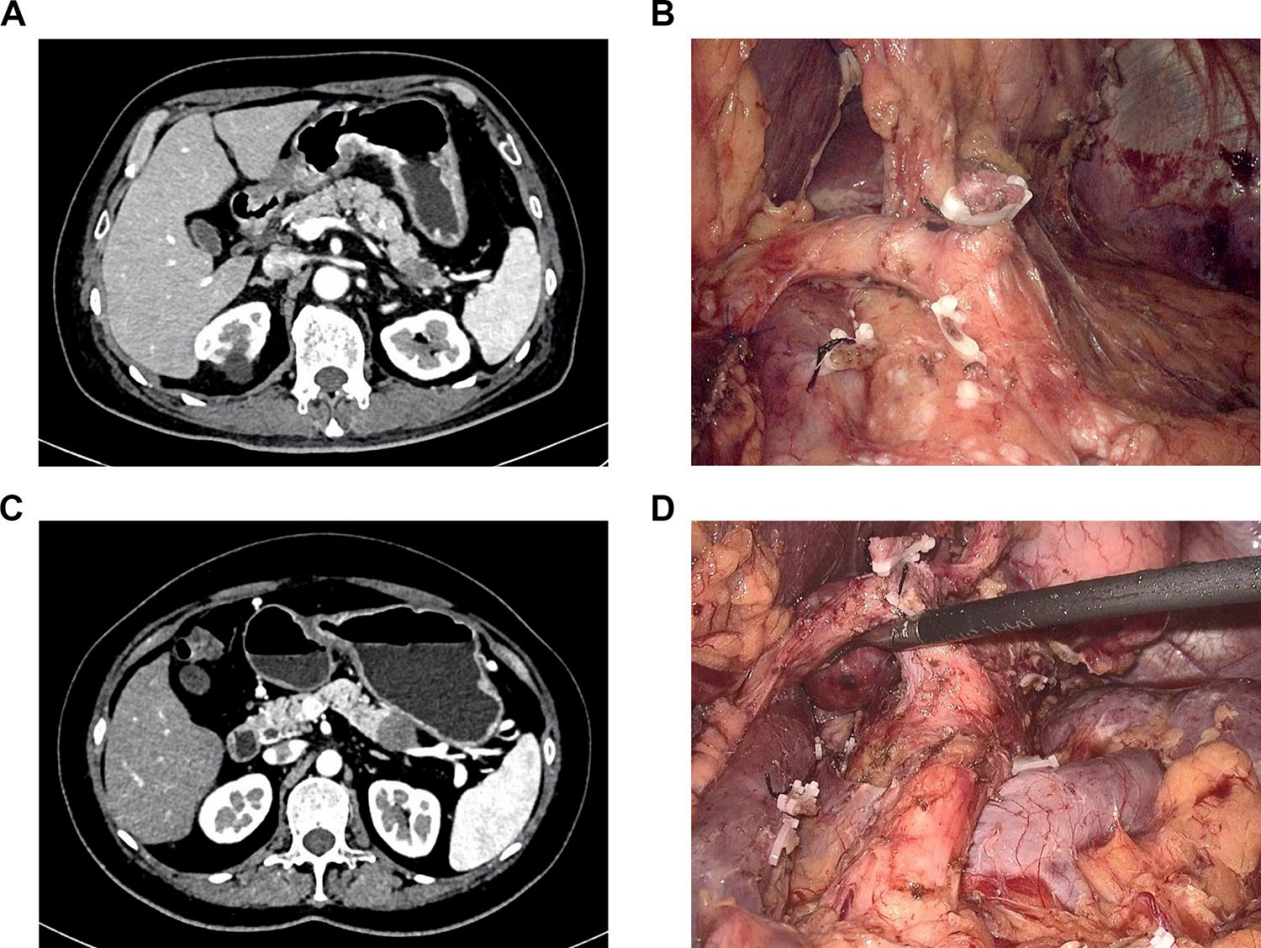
Preoperative CT images of left-sided PDAC meeting the selection criteria and the extent of surgical resection under laparoscopy. A and B Preoperative CT images (A) of a pancreatic tail cancer meeting the selection criteria and the corresponding surgical field after laparoscopic distal pancreatectomy (B); C and D: Preoperative CT images (C) of a pancreatic tail cancer meeting the selection criteria and the corresponding surgical field after laparoscopic radical antegrade modular pancreatosplenectomy (D). *CT* Computed tomography, *PDAC* pancreatic ductal adenocarcinoma
